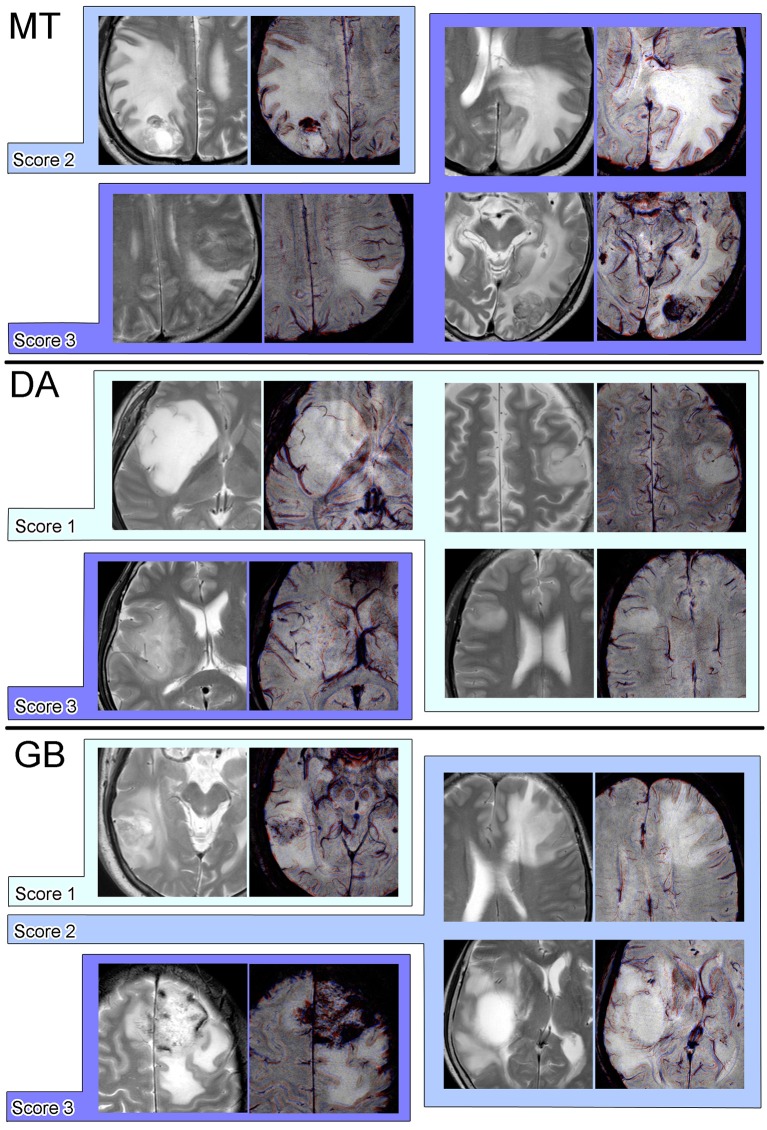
Examples of pairs of T2-weighted and Color PADRE images with scores for SWM on Color PADRE for each tumor type.
